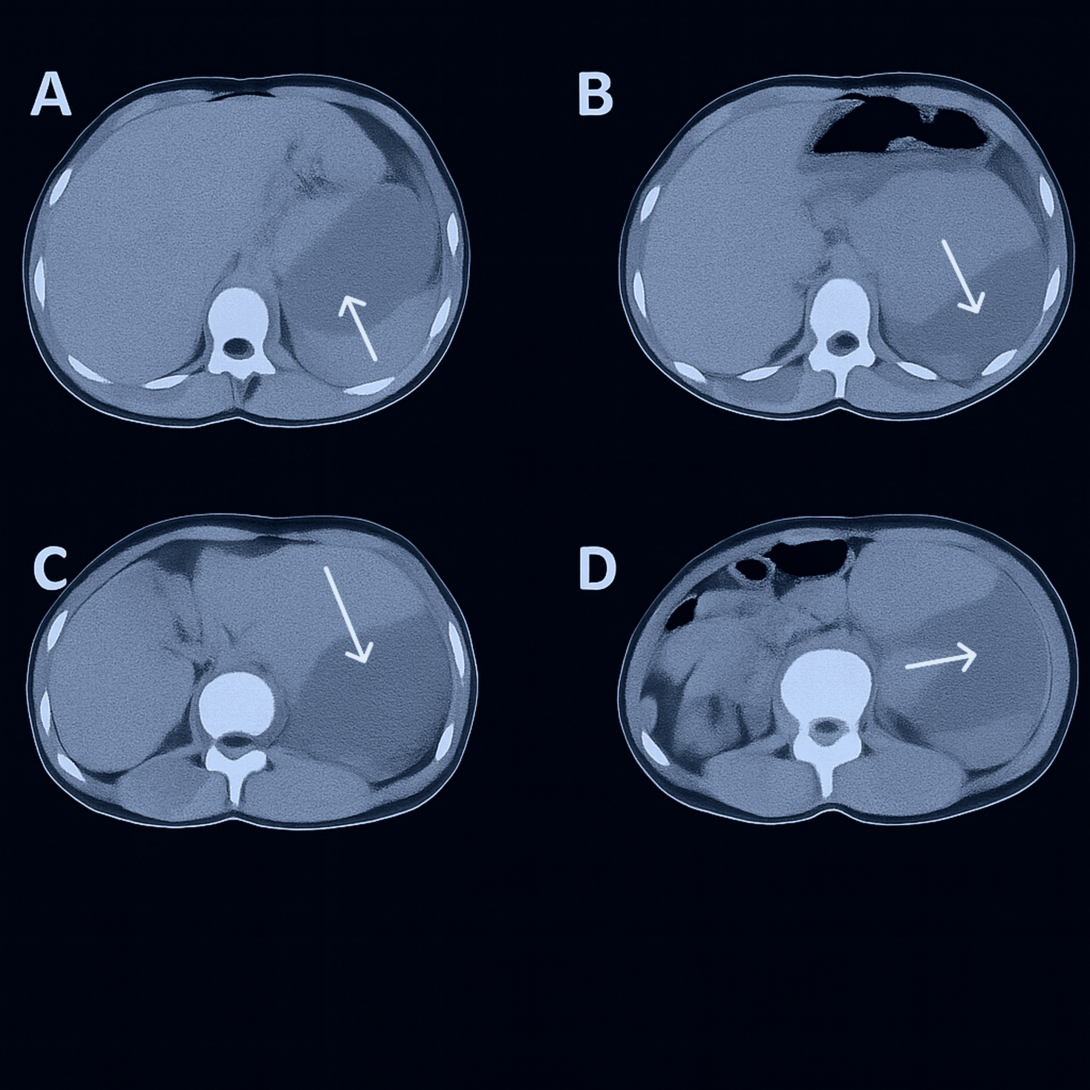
CT urogram axial view Panels A-D: Sequential axial cuts demonstrate significant left hydronephrosis with perinephric fluid collection and contrast extravasation (indicated by arrows) consistent with spontaneous rupture of the renal collecting system. These findings were critical in confirming the diagnosis and guiding surgical management.
